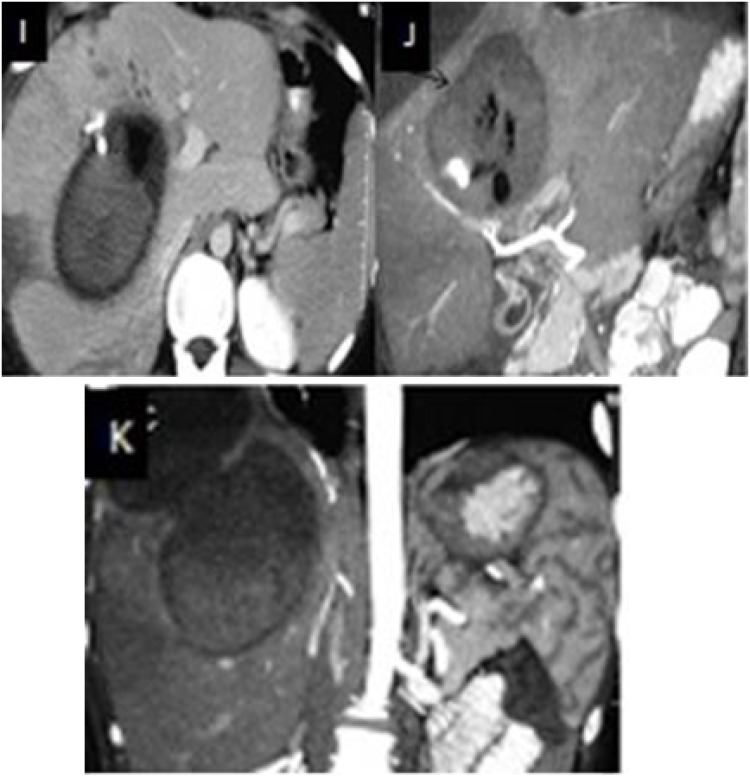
I, J, K) Axial contrast enhanced computed tomography (CECT) scan in arterial phase and coronal reformatted images from liver show pseudo-aneurysm in right hepatic artery (arrow) with infected liver hematoma containing gas density and also sub-capsular hematoma
